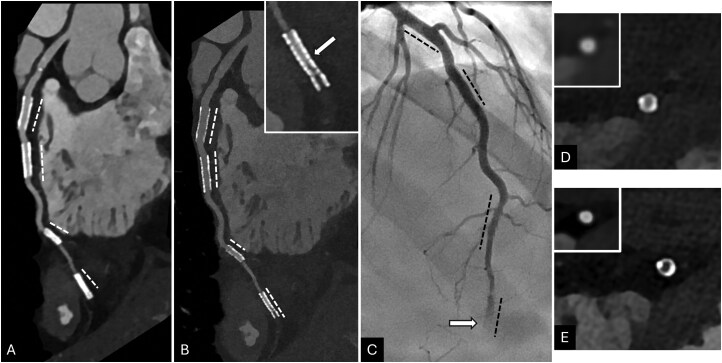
A 36-year-old female underwent PCD-CT coronary angiography examination that was reconstructed with high-resolution parameters with Bv56 kernel. 2-mm slice thickness and 1024 × 1024 matrix (B, D, and E); and parameters mimicking the maximum resolution available on EID-CT with Bv40 kernel, 6-mm slice thickness, and 512 × 512 matrix (A; and insets in D and E). The images demonstrating a left anterior descending (LAD) artery with 4 stents (dashed lines in A, B, and C) with occlusion involving the lower third of the fourth stent (white arrows in B inset and C). The EID-CTA like reconstructions fail to show the lumen in the third and fourth stents (less than 3 mm, A and inset D) and additionally the occlusion (A, and inset E ) that was apparent in high-resolution reconstruction (E) compared to the patent lumen at the proximal part of the stent (D). Abbreviations: CTA, CT angiography; EID, energy-integrating detector; PCD, photon-counting detector.
